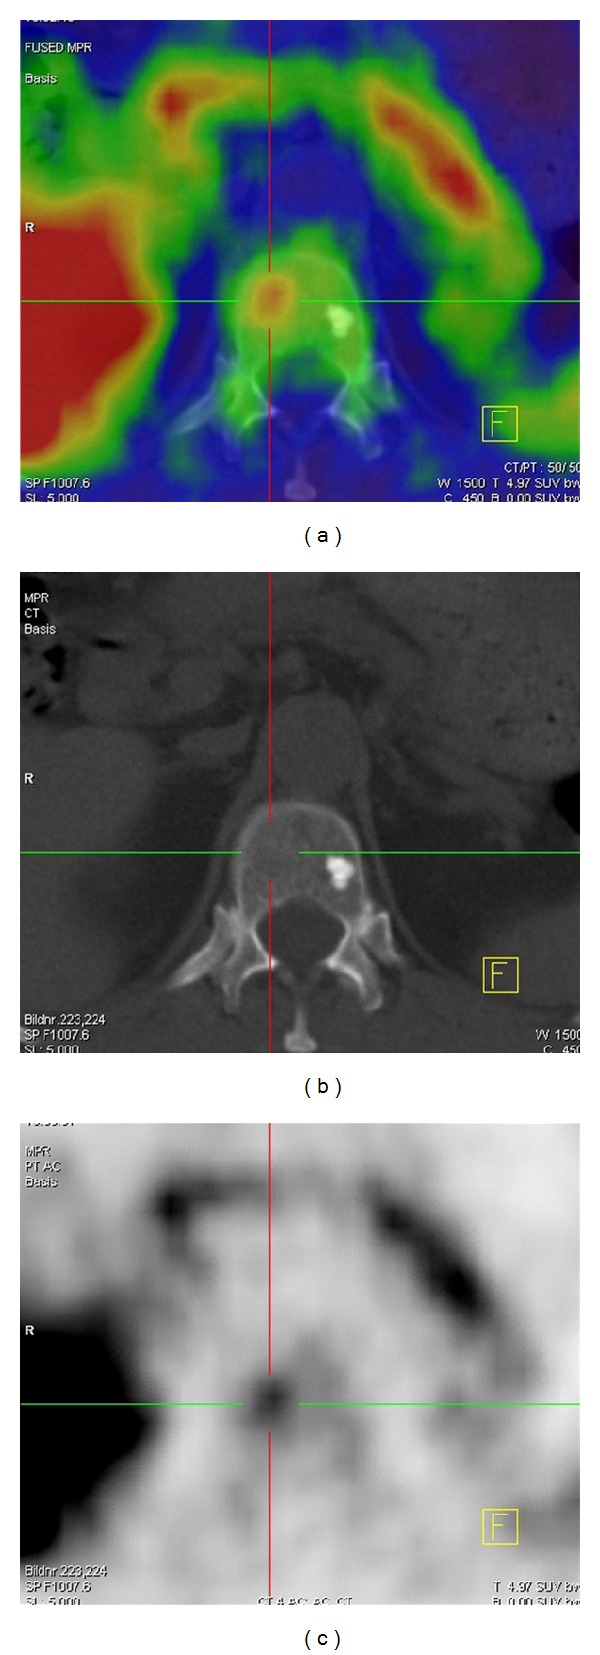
Axial planes of ^18^F-FECH-PET/CT in lower thoracic spine show intensive choline uptake ((a), (c)), attributed to bone marrow involvement without morphological alteration (b). In contrast, in the neighborhood there is an osteoblastic metastasis showing a negative choline uptake.
